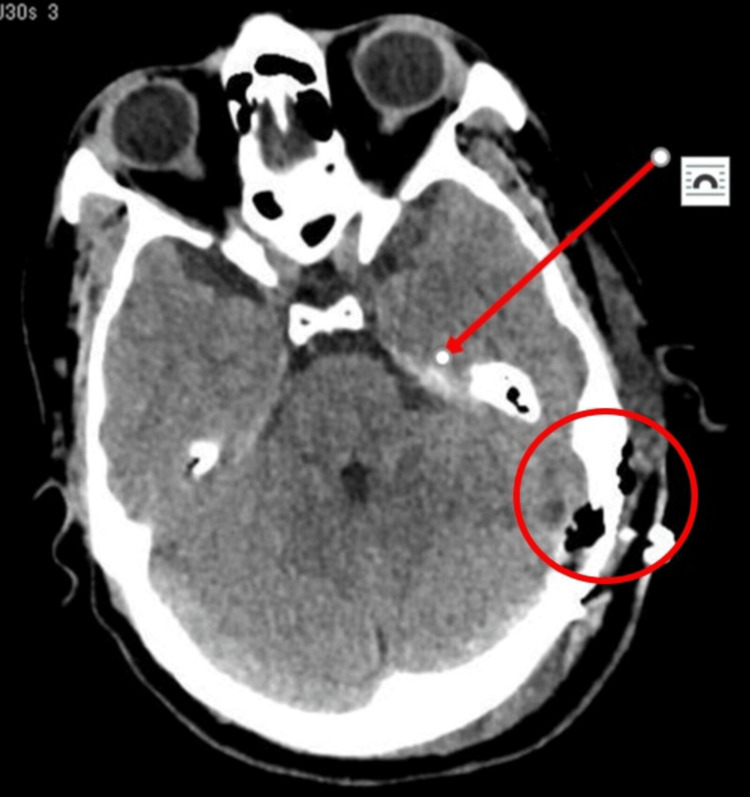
Axial CT scan of the head demonstrating the surgical incision site (red circle) and postoperative changes at the operative site with a focal hyperdensity (red arrow)
